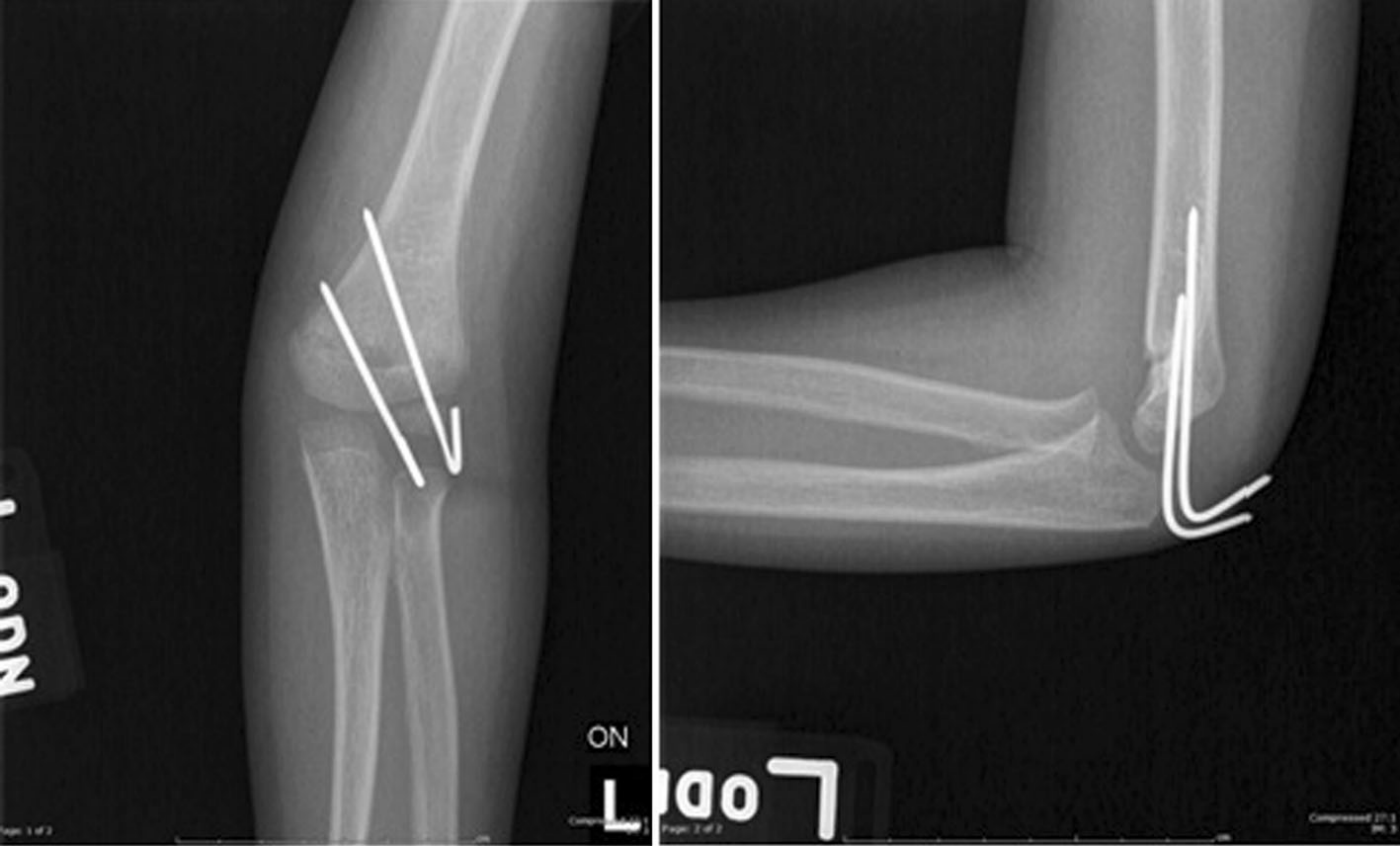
Example of radiograph at time of pin removal for a patient whose immobilization was extended based on surgeon interpretation of radiographic findings
